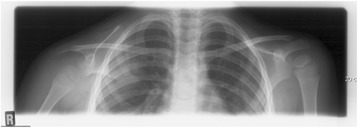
At initial presentation, the radiographs showed an inferior subluxation of the right shoulder
